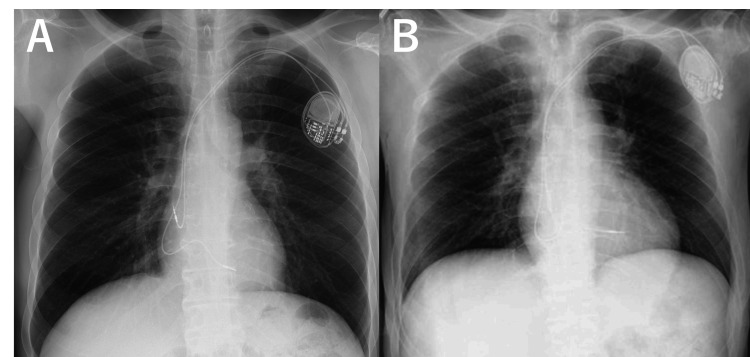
Chest radiographs (A) Taken one year before the current presentation. (B) Taken at the time of the current presentation, showing pulmonary congestion, which may reflect cardiac tamponade. In both radiographs, the pacemaker lead appears markedly bent.
